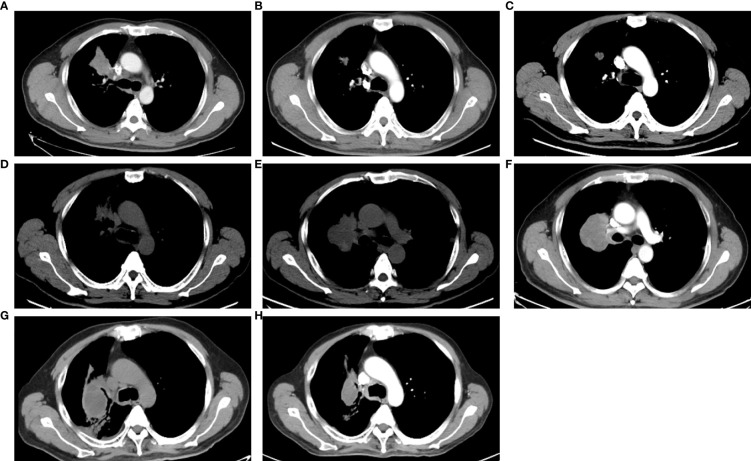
The images of CT scans with different treatments. (A) CT scan of basic examination before first-line erlotinib. (B) CT scan during the first-line erlotinib treatment. (C) CT scan at the progression of first-line erlotinib. (D) CT scan at progression of second-line osimertinib. (E) CT scan at the progression of chemotherapy. (F) CT scan at progression of afatinib plus nimotuzumab. (G) CT scan at the progression of immunotherapy. (H) CT scan during combination therapy of irinotecan, nimotuzumab, and dacomitinib.
